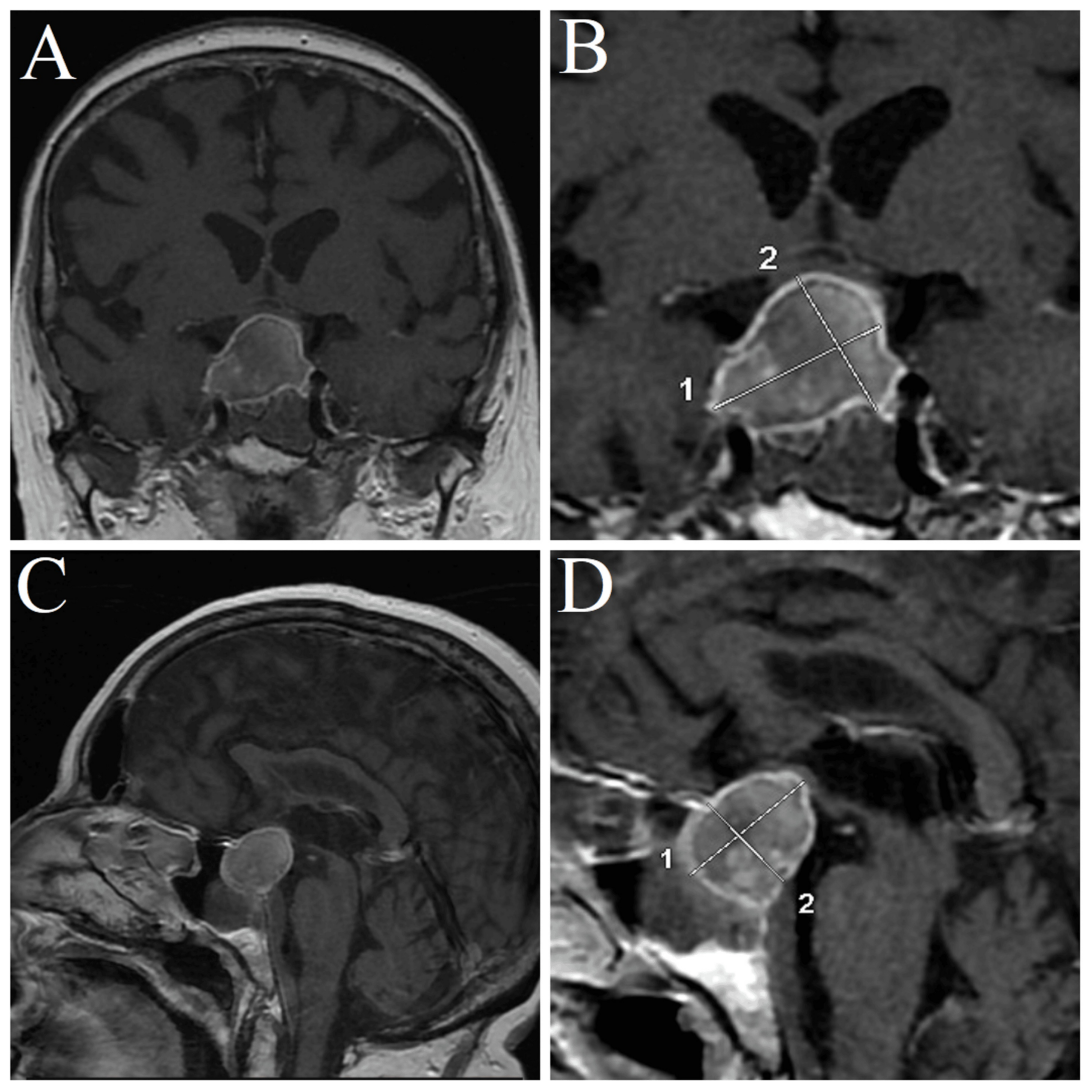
MRI results. (A) MRI in the coronal plane demonstrating pituitary macroadenoma with apoplexy and compression of the optic chiasm and the cavernous sinus bilaterally. (B) Zoomed in on the adenoma. 1 = 29.2 mm, 2 = 24.5 mm. (C) MRI in the sagittal plane demonstrating pituitary macroadenoma with apoplexy, with compression of the optic chiasm. (D) Zoomed in on the pituitary adenoma. 1 = 27.6 mm, 2 = 20.1 mm.
